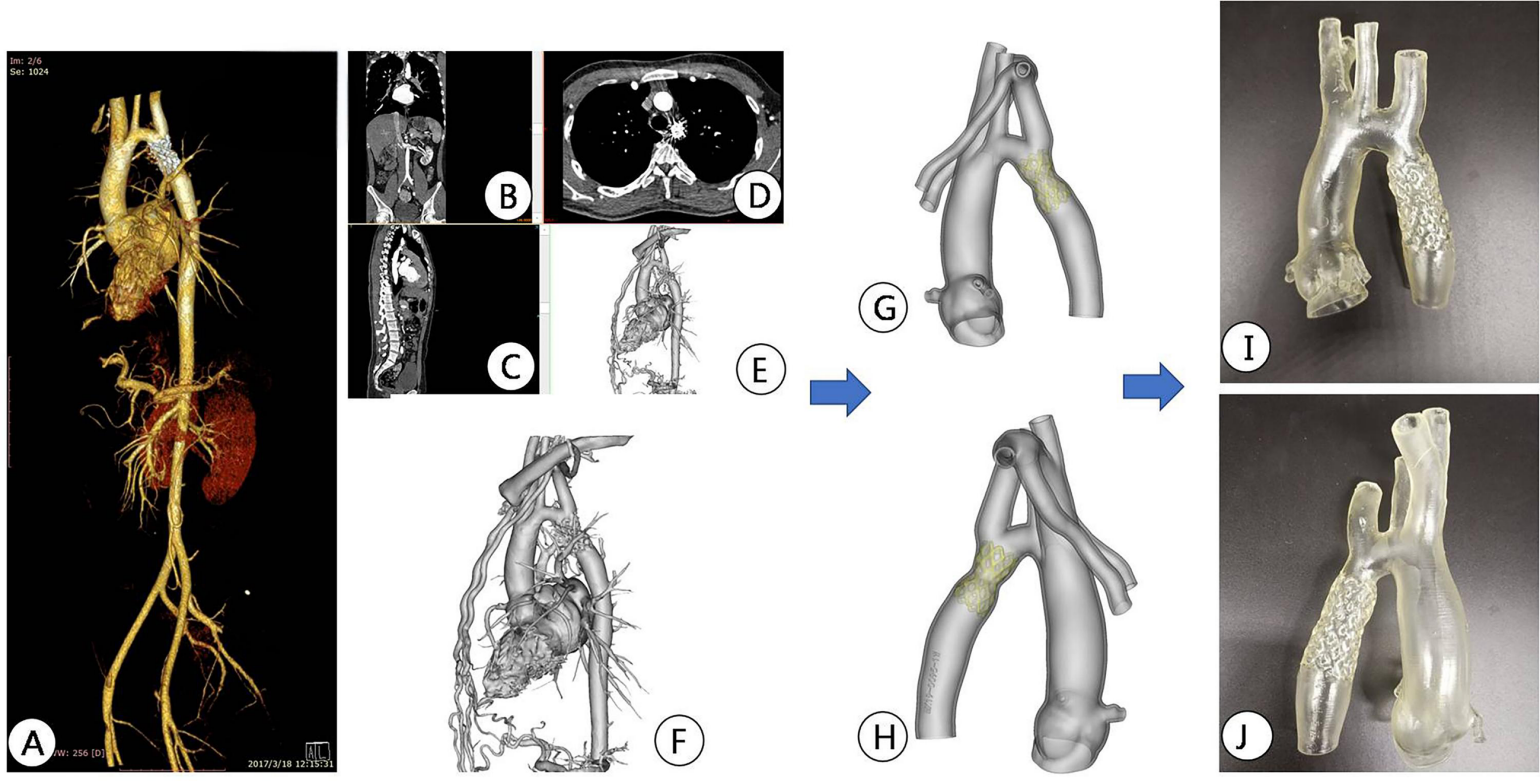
CT image reconstruction and 3D printing model after aortic coarctation surgery. (A) Postoperative aortic CTA reconstruction imaging showed stent position; (B–D) CTA images were imported into Mimics software and displayed different sections; (E,F) reconstruction images after stent implantation; (G,H) reconstructed images were segmented and modified for subsequent 3D printing; (I,J) 3D printed models after stent implantation.
